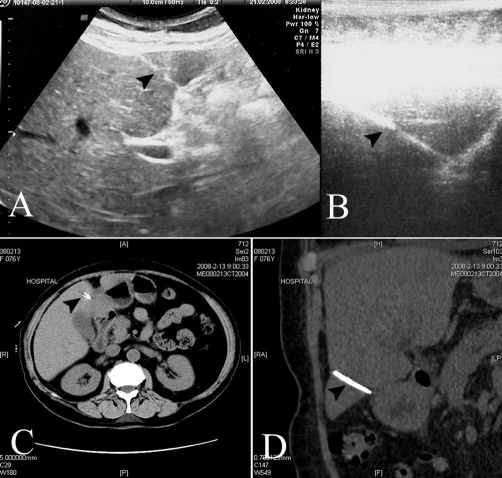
The sewing needle in ultrasound examination and computerized tomographic scan. A: Ultrasound showed a 3.5 cm long needle-like metal object in left lobar of the liver, the arrow points to the needle; B: Partial magnification of the needle in panel A; C: Computerized tomographic scan validated the metal object as a SN, the arrow points to the pore in the needle; D: Reconstructed computerized tomogram of the needle in liver.
